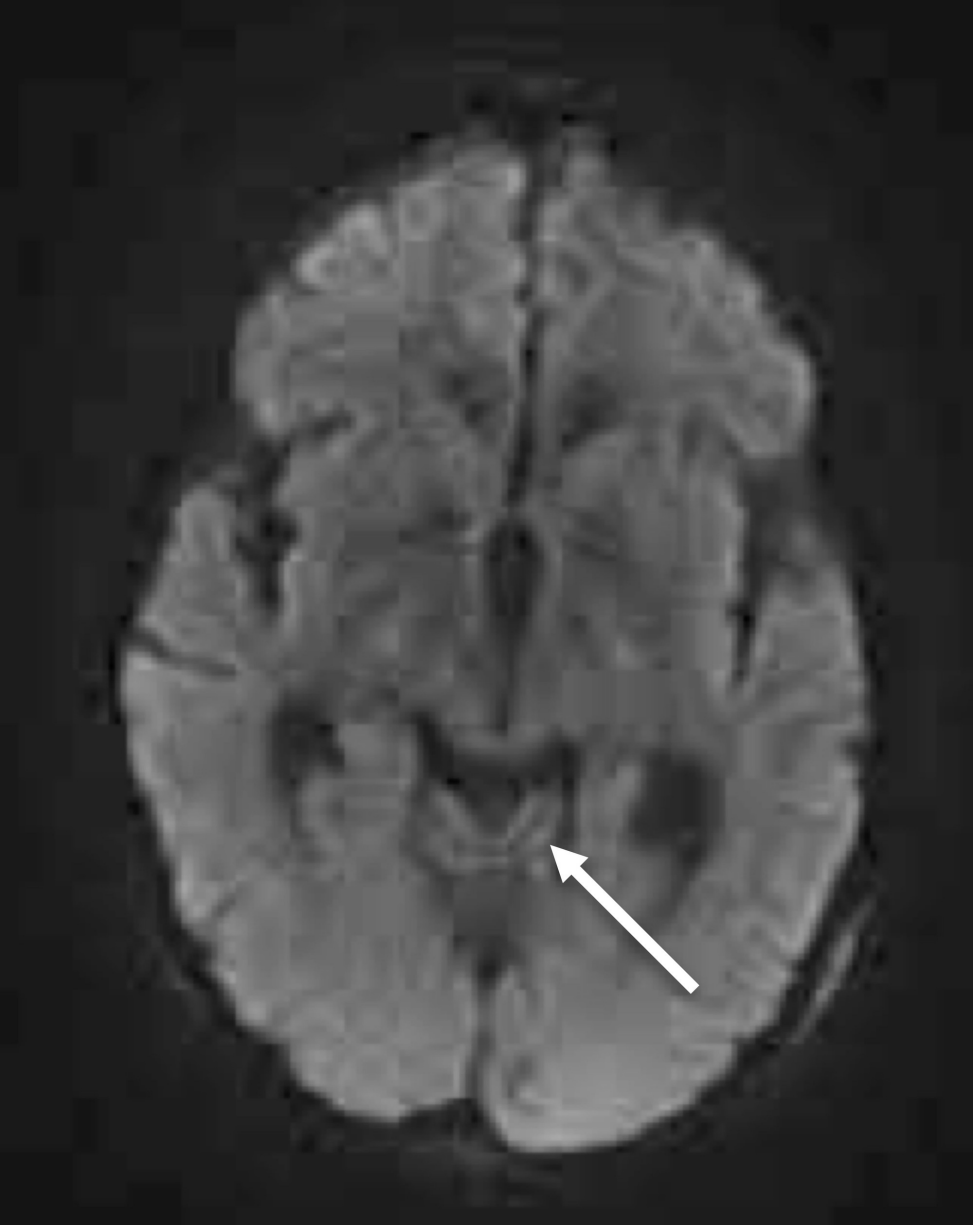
Axial DWI of the head Axial DWI of the head at the level of the cerebellar peduncles shows no restriction in the cerebellar vermis (arrow)
